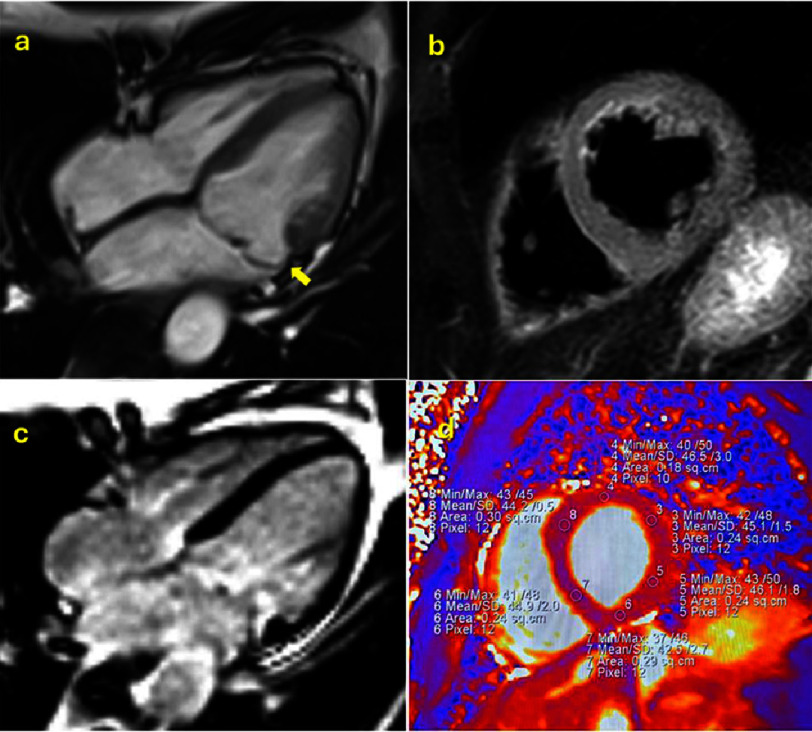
Patients were evaluated by 1.5 T magnetic resonance for volumes, function and morphological study. (a), traditional T2-weighted sequences (b), native T2 mapping (d) and LGE sequences (c); the inclusion criteria were the presence of mitral annulus disjunction (a, yellow arrow), the absence of LGE (c) and the normal myocardial signal in T2-weighted sequences (b) and non-hemodynamically significant mitral regurgitation.
